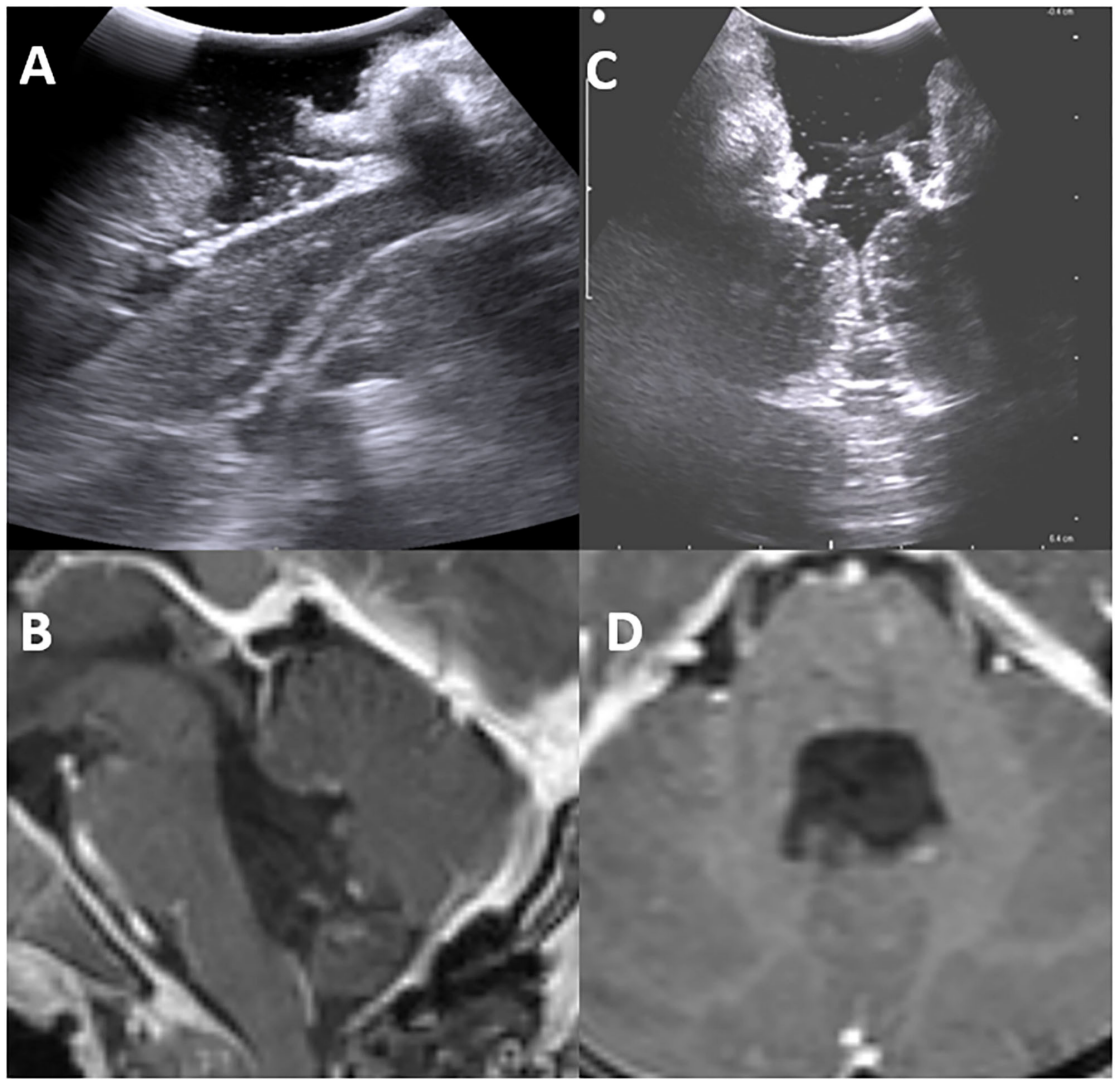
Post-operative intraoperative ultrasound images in sagittal and axial view showing total resection of the lesion (A,C) in concordance with postoperative MRI -TIWI in sagittal and axial views (B,D).
